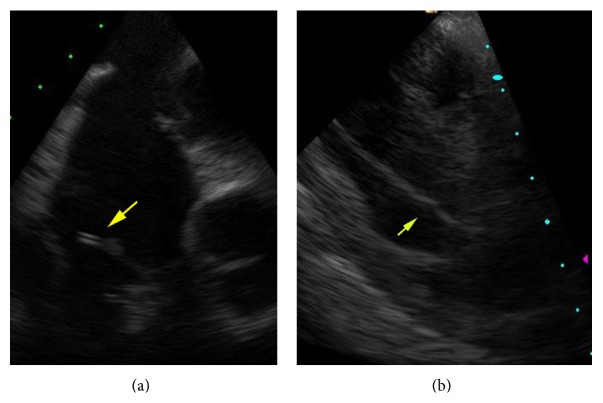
(a) Intracardiac ultrasound-dislodged catheter (arrow) is extending across the tricuspid valve. (b) Intracardiac ultrasound-dislodged catheter (arrow) is seen in the right ventricle.
